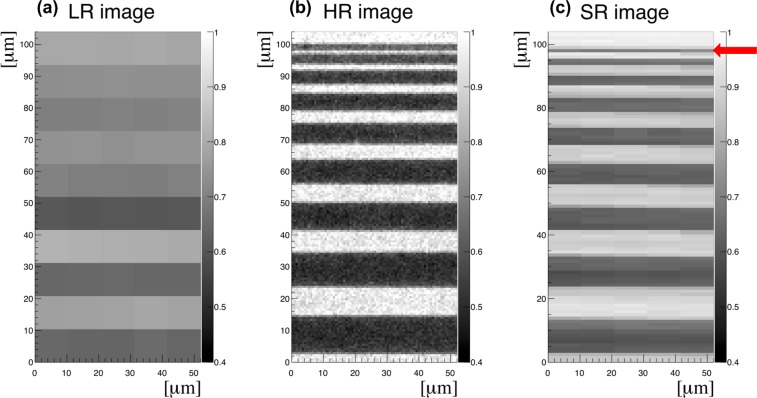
Super-resolution X-ray imaging of a resolution test pattern: (a) low resolution (LR) image (pixel size: 10.4 µm) formed from a high resolution HR image by binning, (b) high resolution (HR) image (pixel size: 0.65 µm) as measured. (c) Reconstructed super resolution (SR) image (pixel size: 1.04 µm) from ten LR images. The smallest feature of 1.5 µm is resolved (indicated by a red arrow). It is seen that the SR image is less noisy comparing to HR image, because SR image was made from ten HR images.
